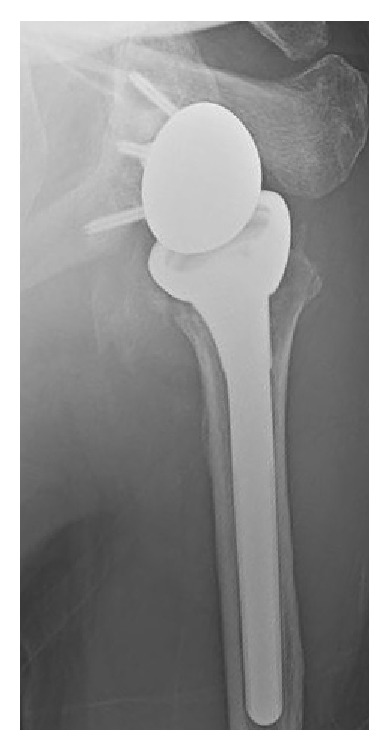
Anterior-posterior radiograph of our patient's reverse total shoulder arthroplasty at three months after implantation.
